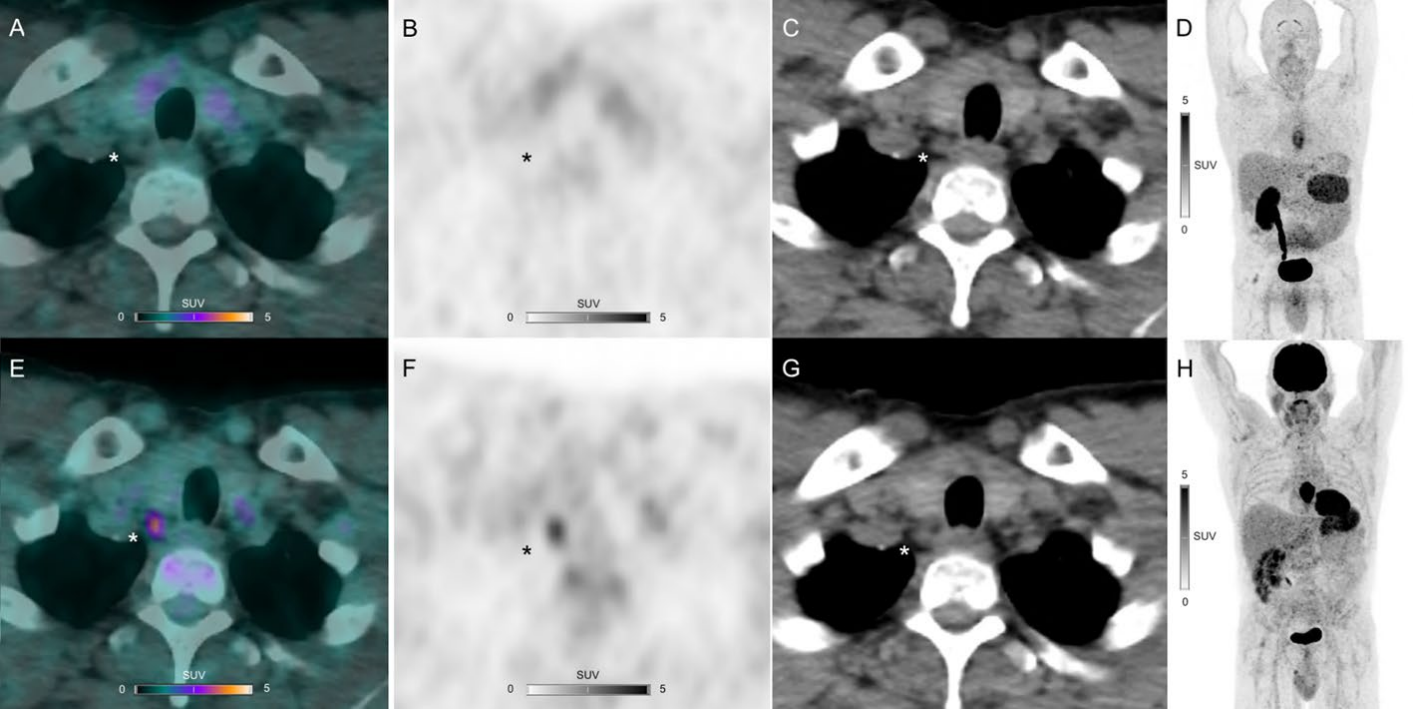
^68^Ga-NODAGA-RGD PET/CT (A), PET (B, D) and CT (C) and ^18^F-FDG PET/CT (E), PET (F, H) and CT (G) views of an upper paratracheal lymph node metastasis (asterisks) showing no increased ^68^Ga-NODAGA-RGD uptake but intense ^18^F-FDG uptake (SUV_max_ 3.9 g/mL) in a 59-year-old participant with esophageal squamous cell carcinoma
